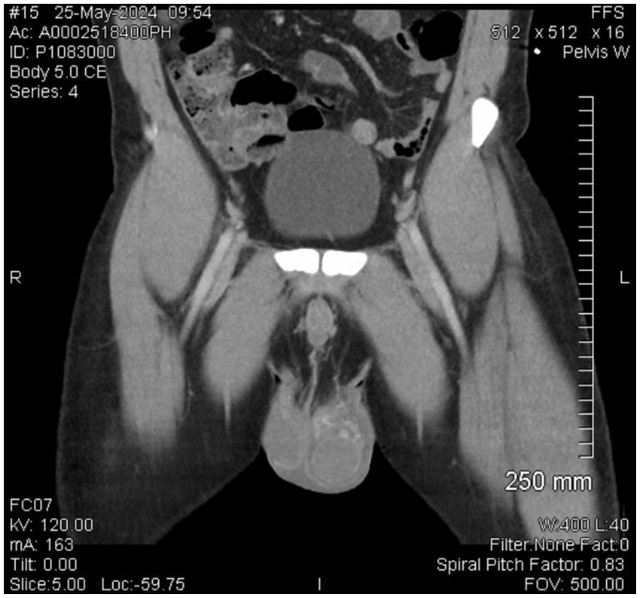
CT scan of the pelvis showing scrotal edema involving both scrotal sacs.
